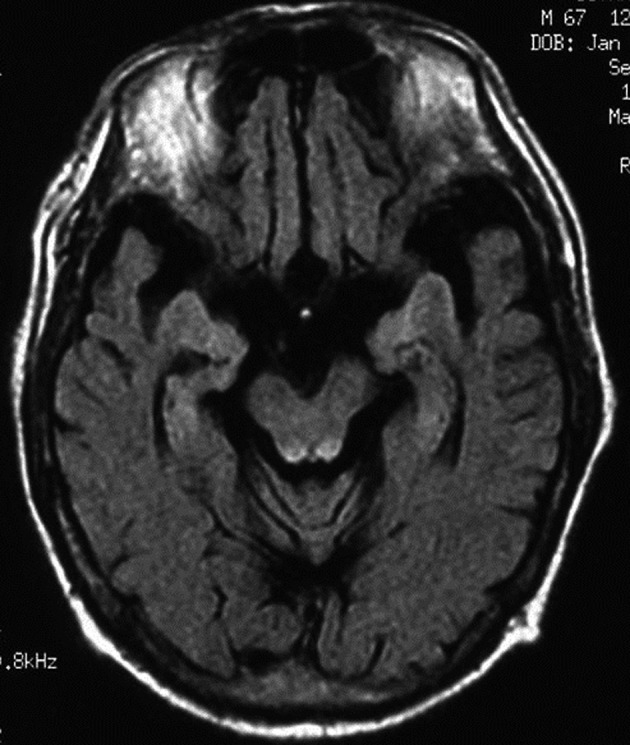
MR imaging (FLAIR) also showed hyperintense lesion of the brain stem.
